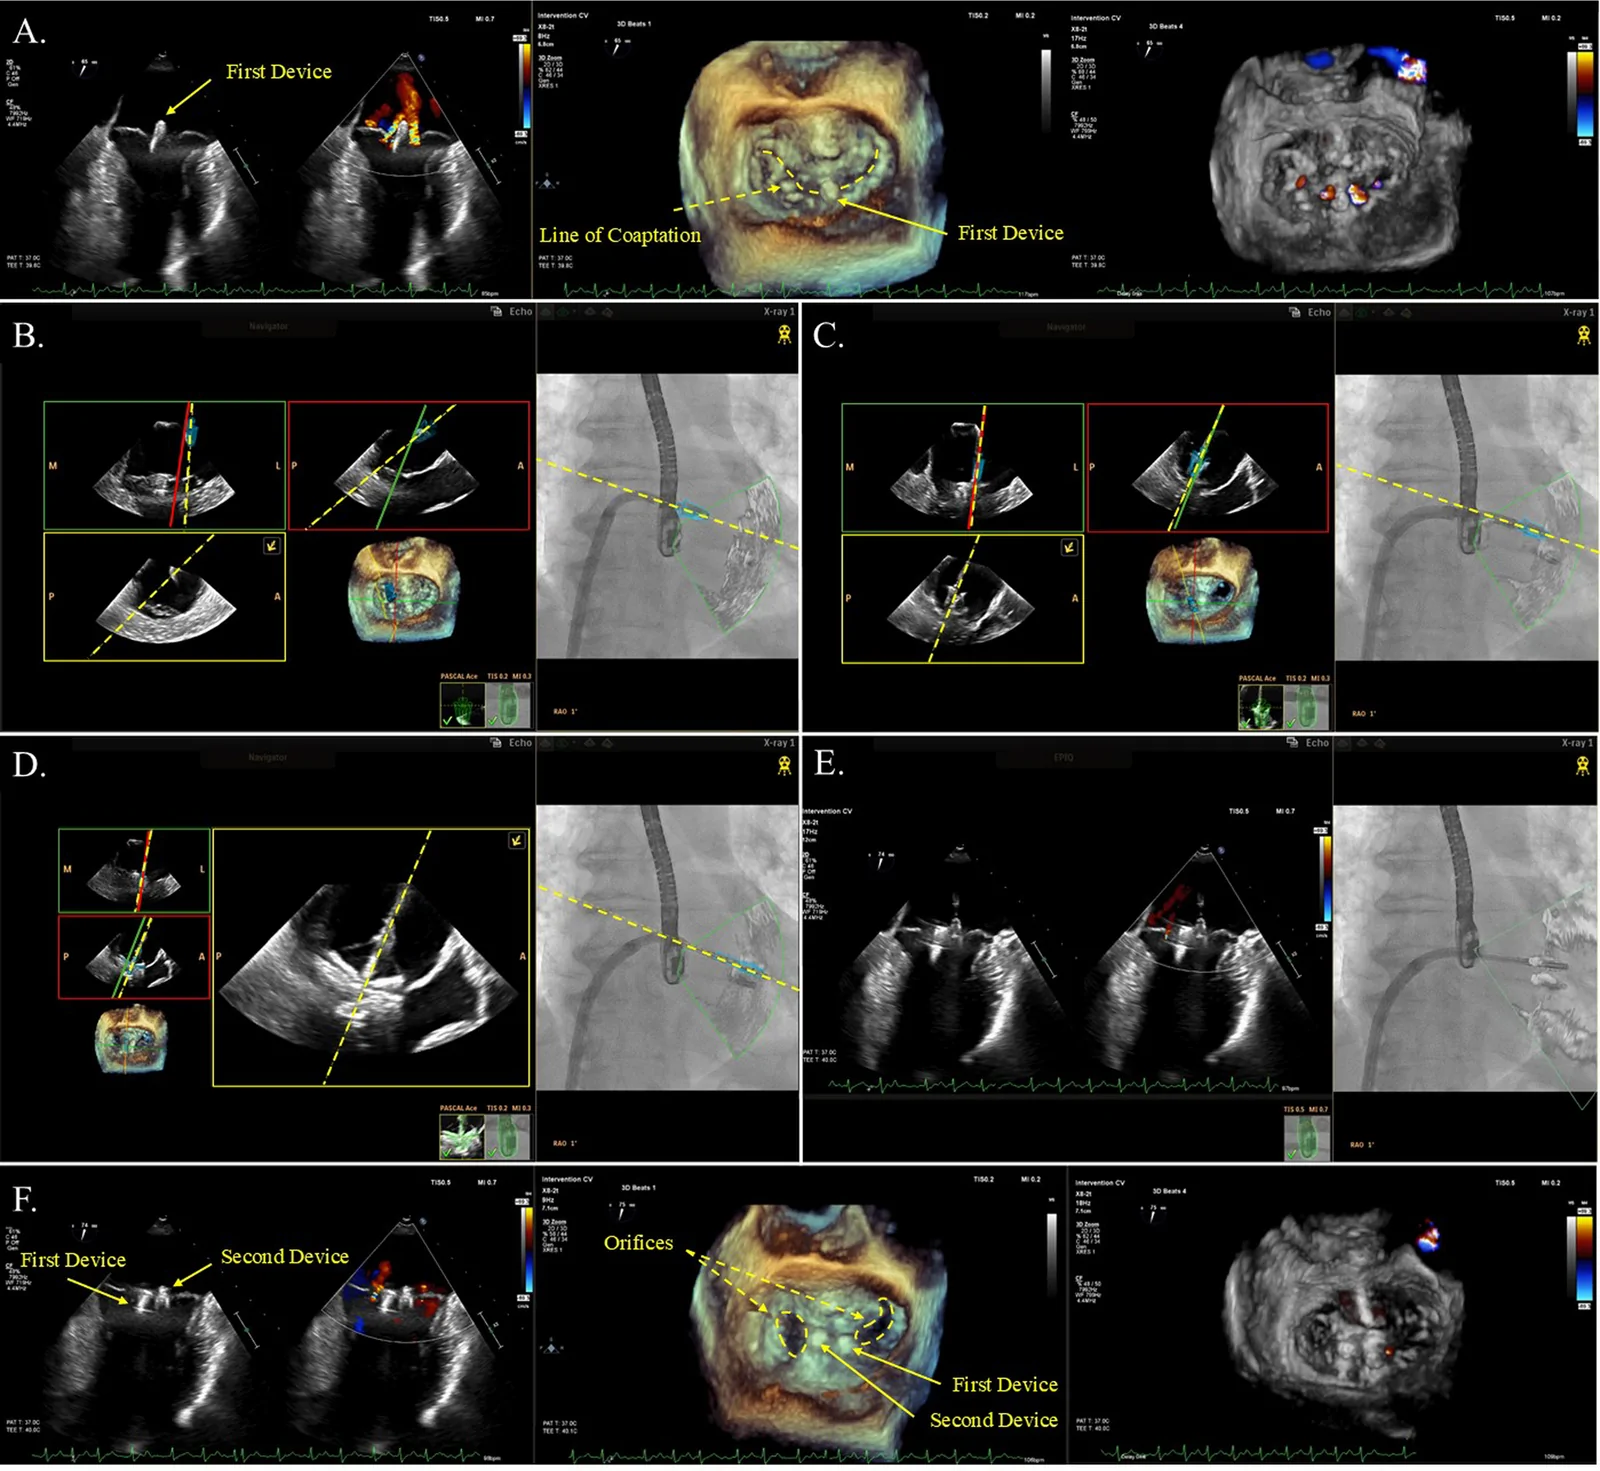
Stepwise echocardiographic guidance during second clip implantation. A The 2D and 3D transesophageal echocardiography (TEE) with color Doppler confirming residual mitral regurgitation (MR) following implantation of the first device, prompting a second transcatheter edge-to-edge repair (TEER) device. B, C Multiplanar reconstruction and DeviceGuide (EchoNavigator SmartVue, Philips Healthcare) views identify the origin of the residual regurgitant jet and defining the optimal position and alignment for the second TEER device relative to the first. D DeviceGuide maintaining continuous real-time visualization of the second TEER device trajectory and alignment throughout closure. E Echocardiography-fluoroscopy fusion imaging supporting precise spatial orientation and positioning of the second TEER device relative to the first implanted clip within the constrained left atrial workspace. F Final postdeployment assessment with 2D and 3D TEE confirming near-complete MR elimination with preserved leaflet mobility
